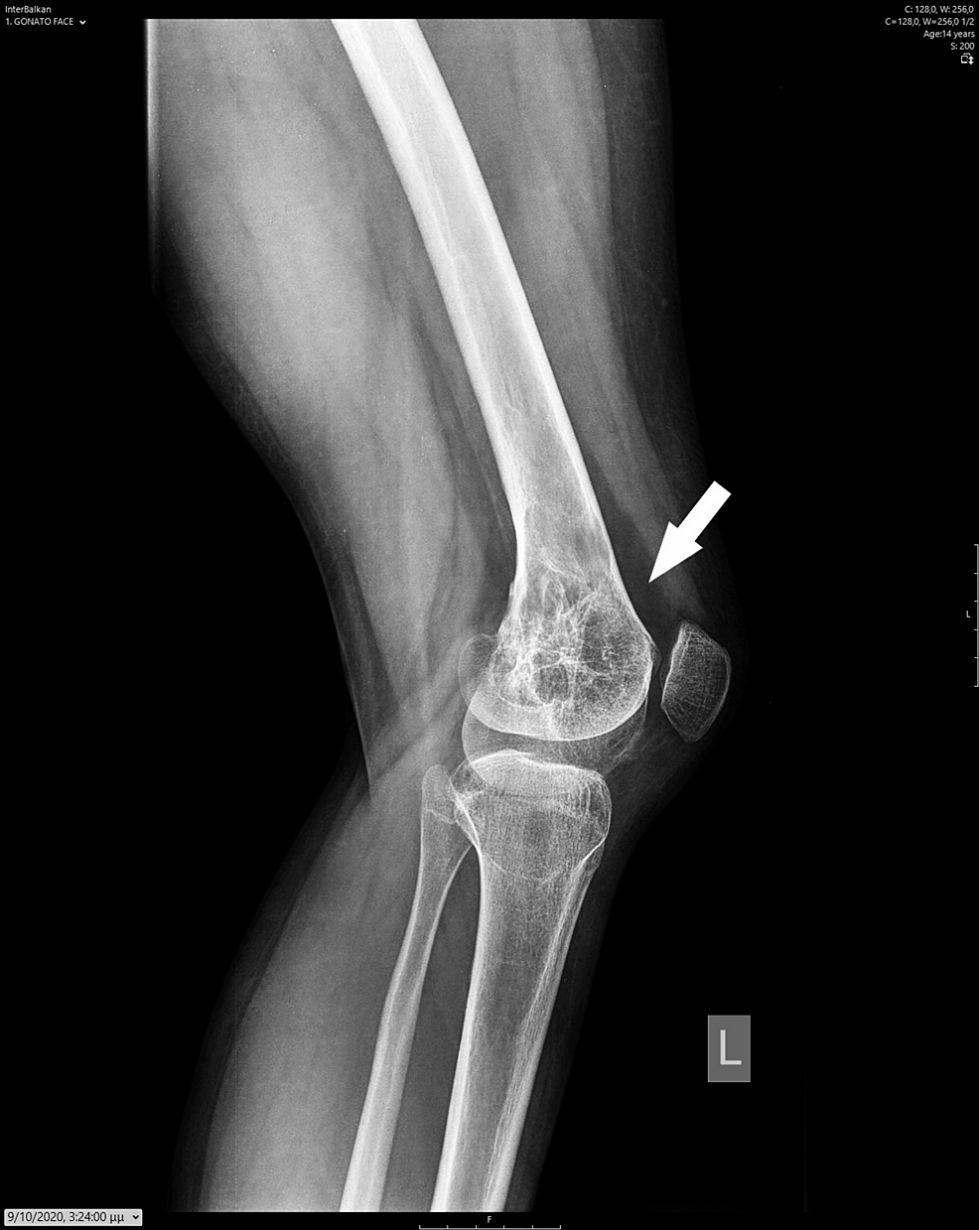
Lateral X-ray image depicts the healed cyst and the restored cortex.
